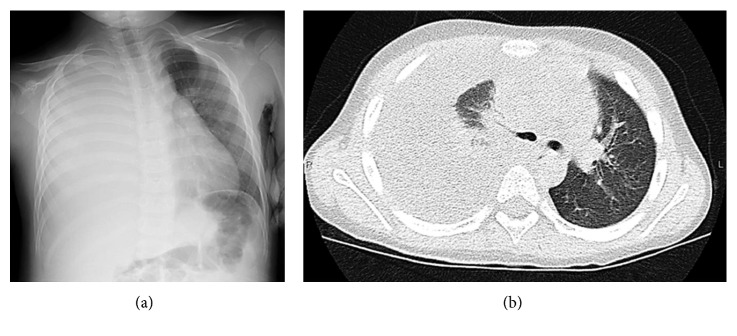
(a) Chest X-ray and (b) chest computed tomography scan of the thoracic region. The images indicate a large pleural empyema of a right pulmonary lesion.
